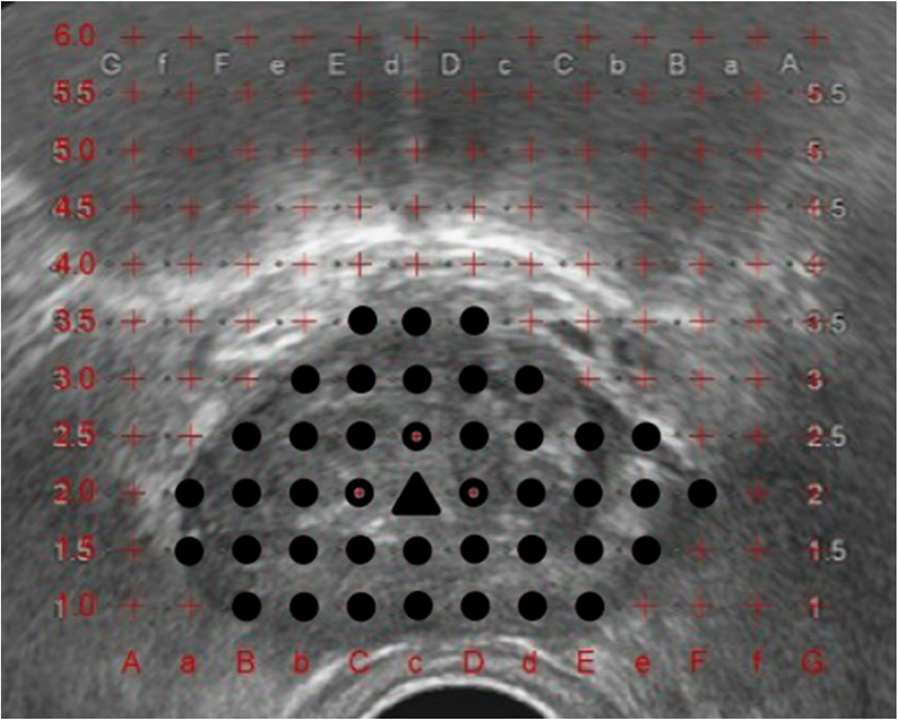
Ultrasound image of the prostate showing the sites for sampling (*black dots*). *Triangle* shows urethra. *Circle* shows sites where sampling was avoided
